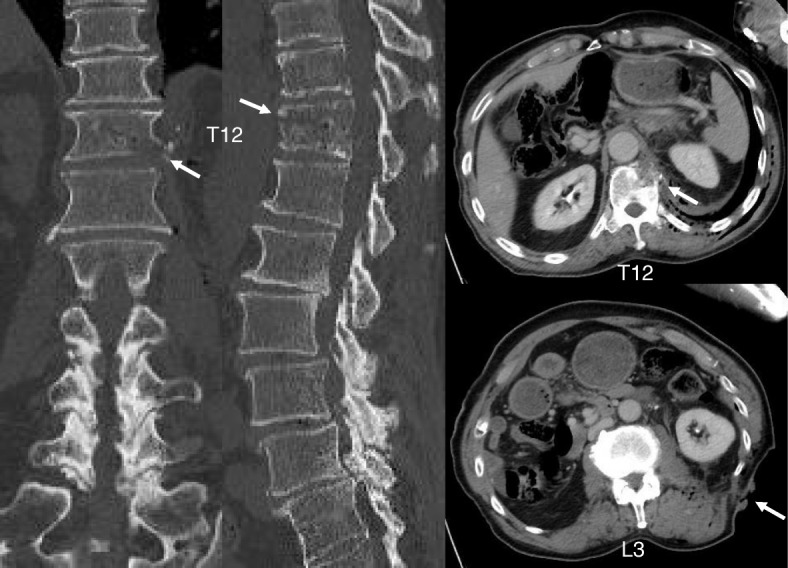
Reconstruction computed tomography showed the fracture of T12 vertebral body. The fracture line passed from the left inferior edge of T12 vertebral body towards the central upper edge of T12 vertebral body (left, middle: fracture line: white arrows). Axial views of computed tomography scan showed the fracture of T12 vertebral body and an abdominal stab wound at L3 level (right upper: anterolateral bone avulsion of the left side of the T12 vertebral body: white arrow, right under: an abdominal stab wound: white arrow)
